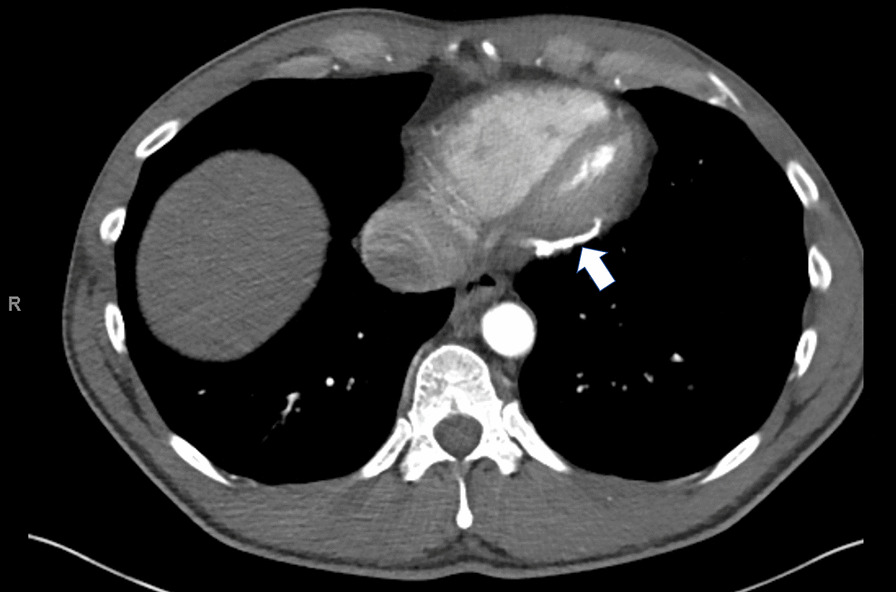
Computed tomography demonstrates pericardial calcification (white arrow)
